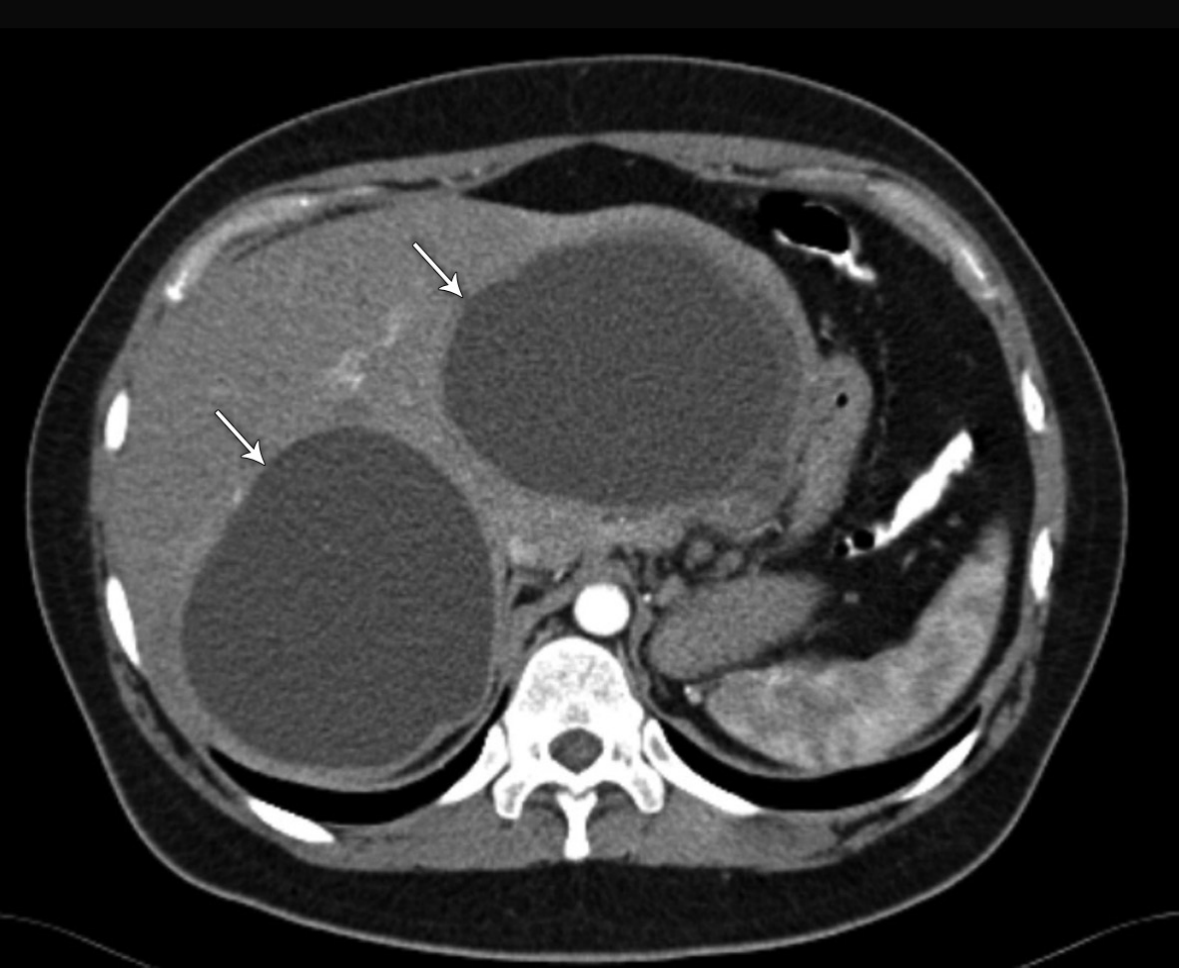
Contrast-enhanced chest computed tomography scan in the axial plane, showing 2 cysts in the liver (arrows).
